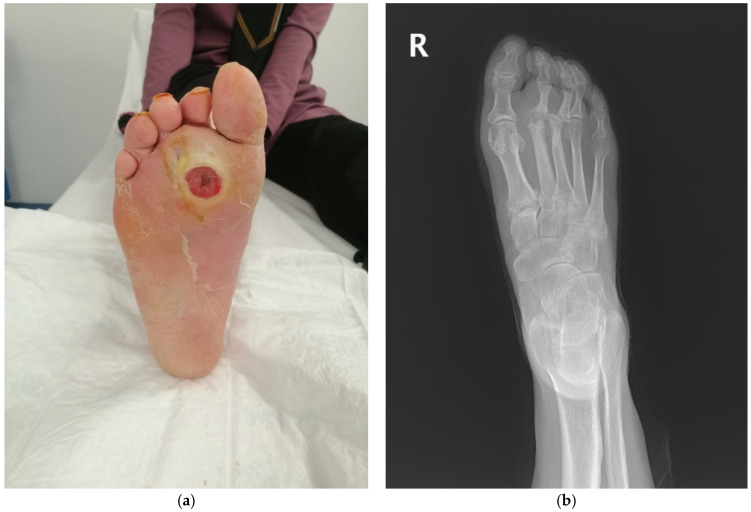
(a): Clinical image of a 62-year-old female patient with a neuropathic diabetic foot ulcer located on the plantar surface. IDSA/IWGDF ulcer classification: Grade 3. (b): Corresponding X-ray image of the same patient showing radiographic findings consistent with osteomyelitis. Osteolytic lesions, cortical erosion, and irregularities are observed in the phalanges and metatarsal bones.
